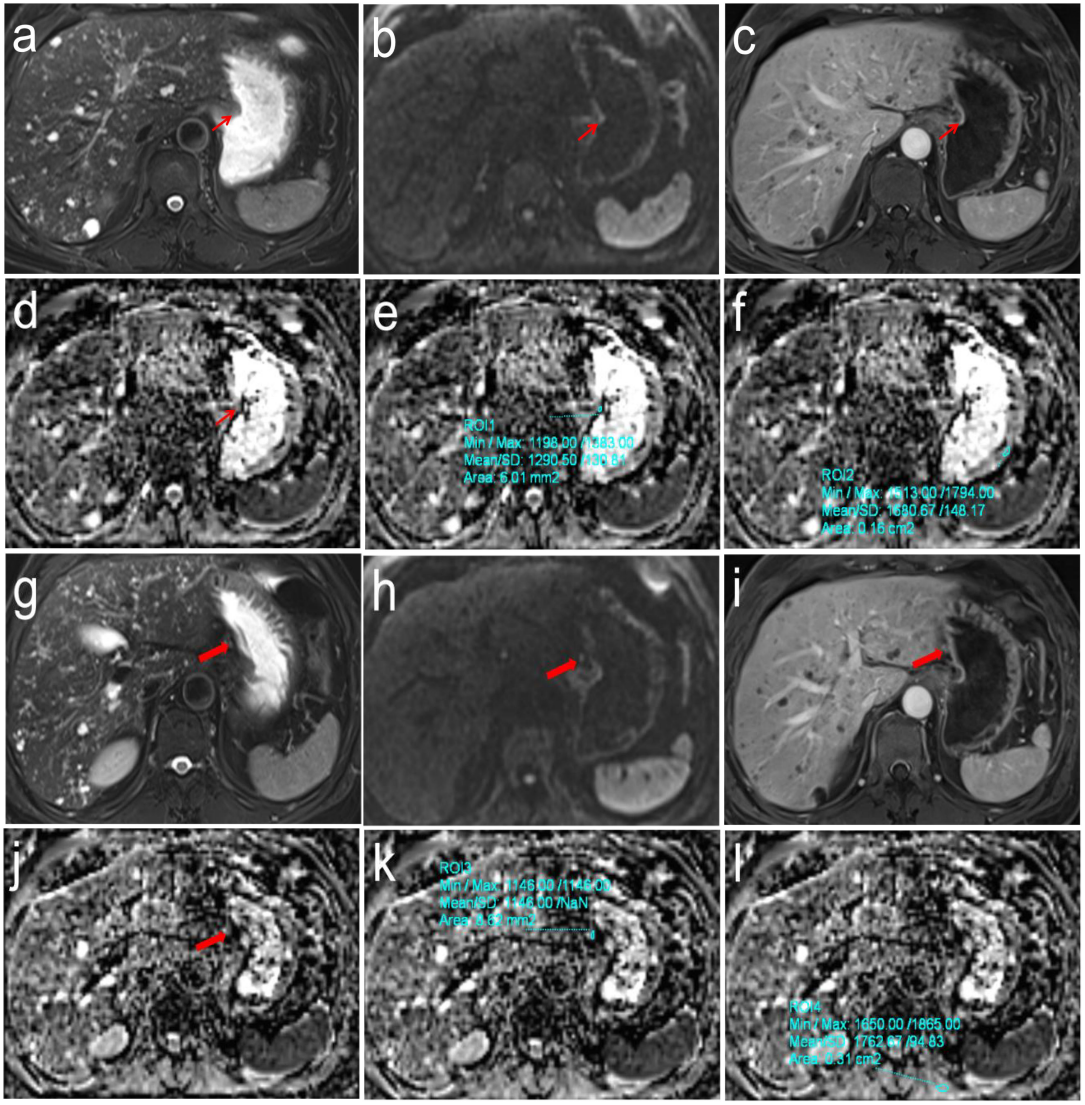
Pathologically confirmed gastric corpus adenocarcinoma (pT1N0M0) in a 62-year-old male. (a-f) T2WI, DWI and DCE images showed the location of primary tumor (Thin red arrow). The ADC values of primary tumor and normal gastric wall of the same axial section were 1.291 × 10^-3^mm2/s and 1.681 × 10^-3^mm2/s, respectively, the correspond rADCT value were 0.768; (g-l) T2WI, DWI and DCE images showed the location of perigastric sentinel lymph nodes (No. 3) (Thick red arrow). The short-axis diameter was about 3.2mm. The ADC values of target sentinel lymph nodes and the left vertical spinal muscle at the same axial section were 1.146 × 10^-3^ mm2/s and 1.763 × 10^-3^ mm2/s, respectively, the correspond rADCLN value were 0.650.
